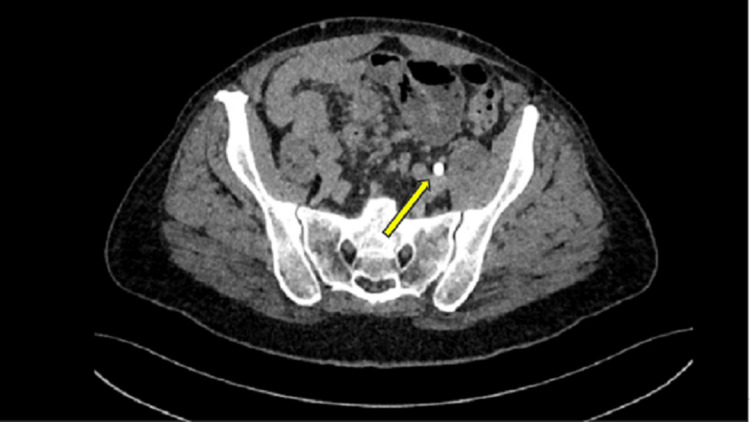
Axial CT with contrast, delayed depiction, with an arrow ​indicating ​the ureter.
